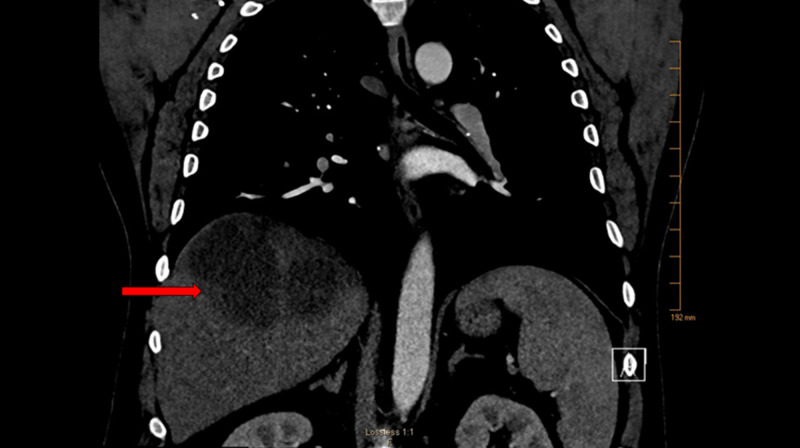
CT chest with contrast revealed large irregular loculated hypoattenuating lesion with rim enhancement in the posterior right hepatic lobe, measuring 8.0 x 12.2 x 8.8 cm, concerning for hepatic abscess
